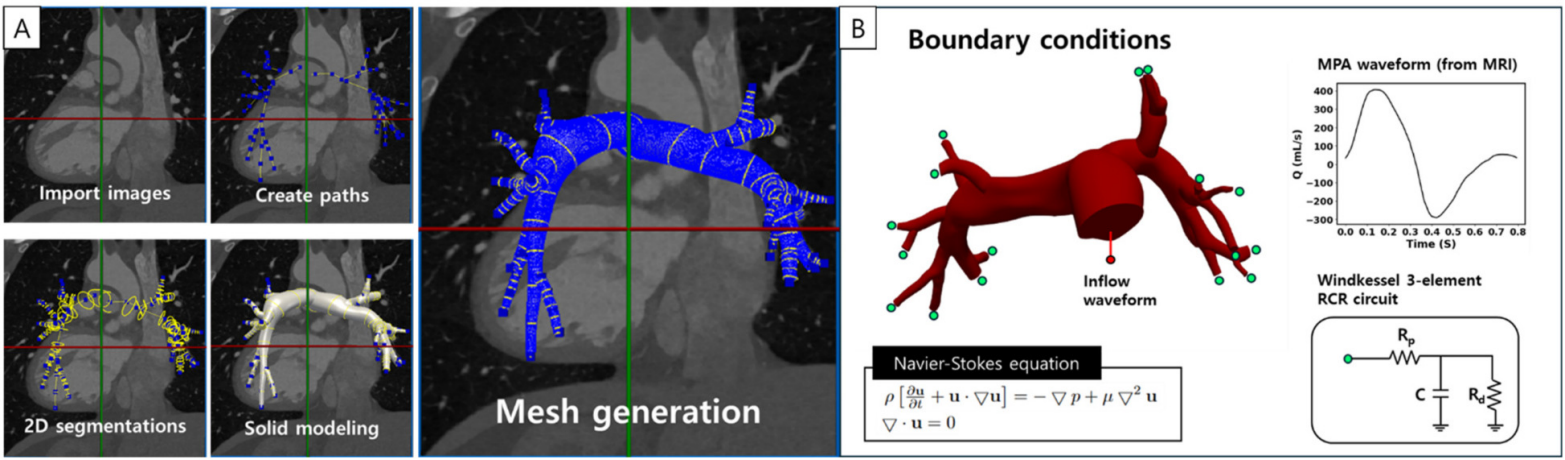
(A) Patient-specific three-dimensional pulmonary artery modeling involves path determination, segmentation, solid model, and mesh generation. (B) Boundary conditions for the pulmonary artery model. The inflow waveform is obtained from magnetic resonance imaging (MRI) of the main pulmonary artery, and outlet boundary conditions are assigned using the Windkessel resistance-compliance-resistance (RCR) model at each outlet. The Navier-Stokes equation consists of the flow velocity u, pressure p, fluid density ρ, and dynamic viscosity μ. MPA, main pulmonary artery.
